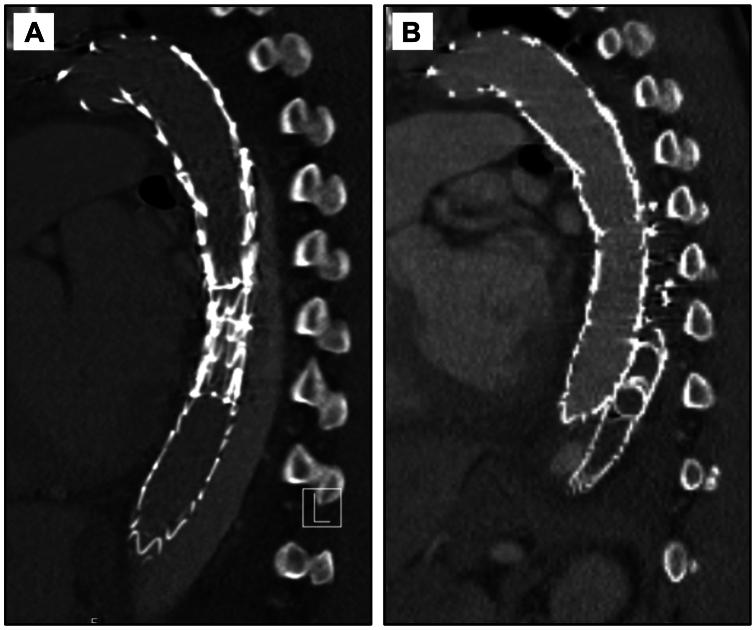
Case 3 computed tomography angiograms. (A) Preoperative image shows extensive endoleak into the false lumen (FL). (B) Three-month postoperative image showing interval resolution of endoleak with thrombosed FL.
